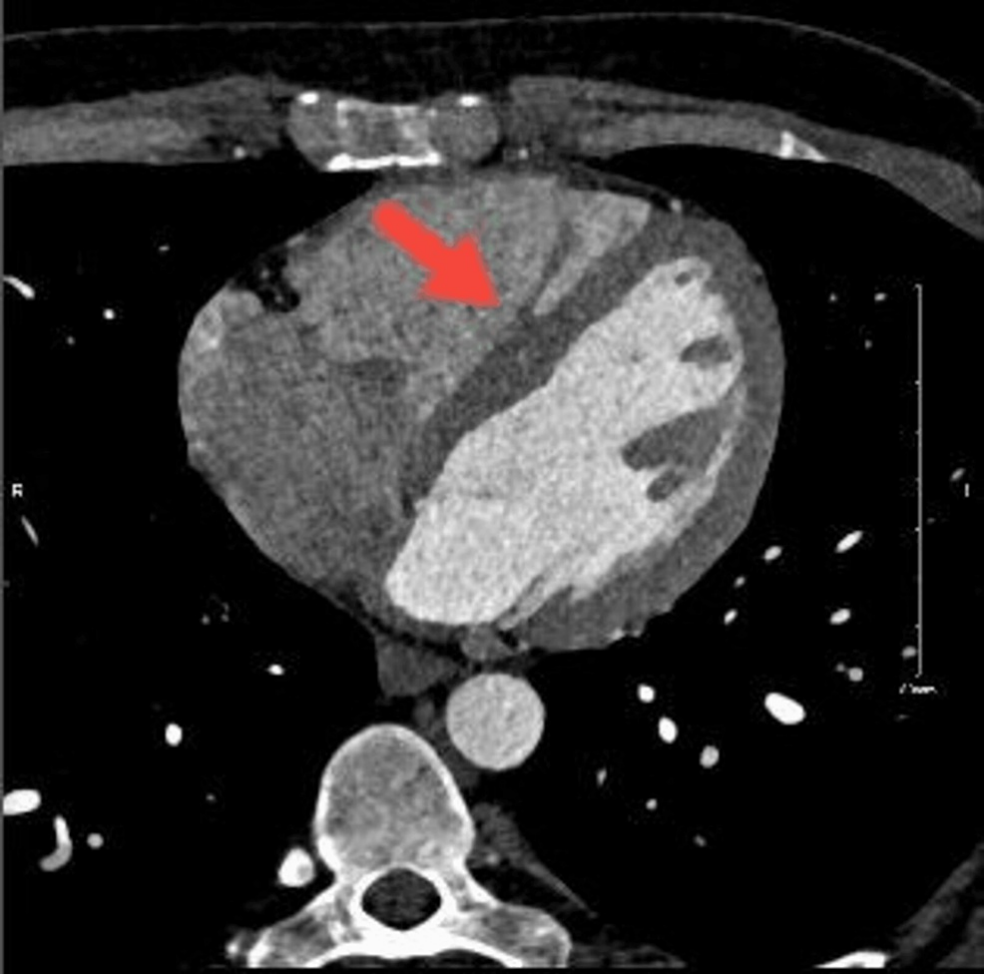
Coronary computed tomography angiography showing the posterior descending artery terminating with a large fistula (arrow) communicating with the left ventricle.
